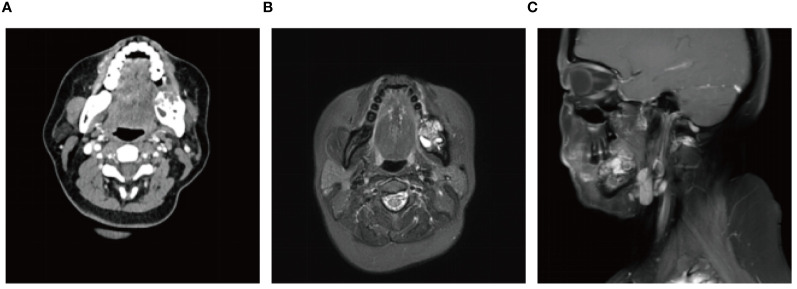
Preoperative maxillofacial imaging. (A) Cross-section plane of the preoperative computed tomography scan; (B) Cross-section plane of preoperative magnetic resonance; (C) Coronal plane of preoperative magnetic resonance.
